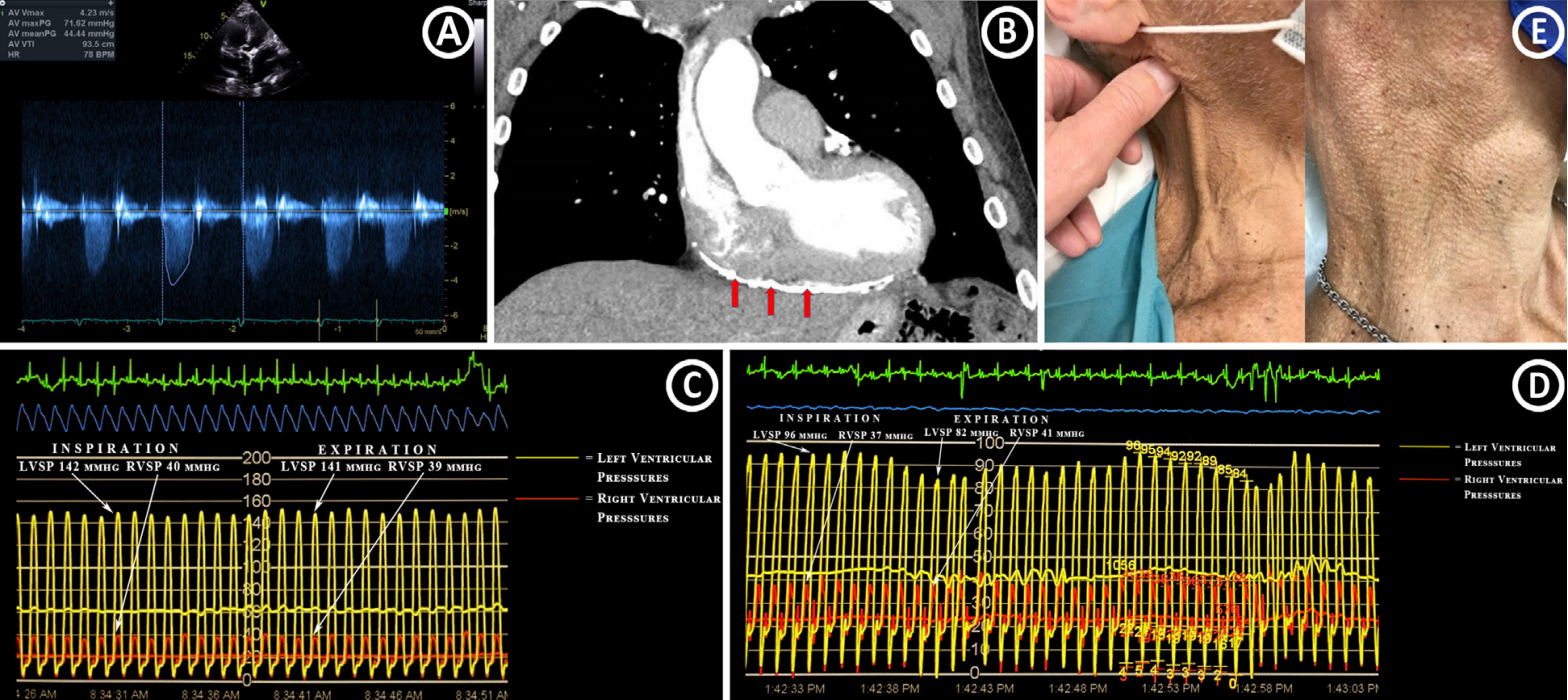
(A) Severe aortic stenosis with a mean gradient of 44 mm Hg on an echocardiogram. (B) A computed tomography scan showing pericardial calcification and thickening (red arrows). (C) Invasive hemodynamic tracing before transcatheter aortic valve replacement (TAVR) showing the absence of ventricular interdependence. (D) Post-TAVR invasive hemodynamic tracing showing the presence (unmasking) of ventricular interdependence. During inspiration, the peak left ventricular systolic pressure is (LVSP) reduced, with a corresponding increase in the right ventricular systolic pressure (RVSP). During expiration, the peak LVSP increased, with a corresponding decrease in the RVSP. (E) Before (left) and after (right) TAVR and pericardiectomy.
